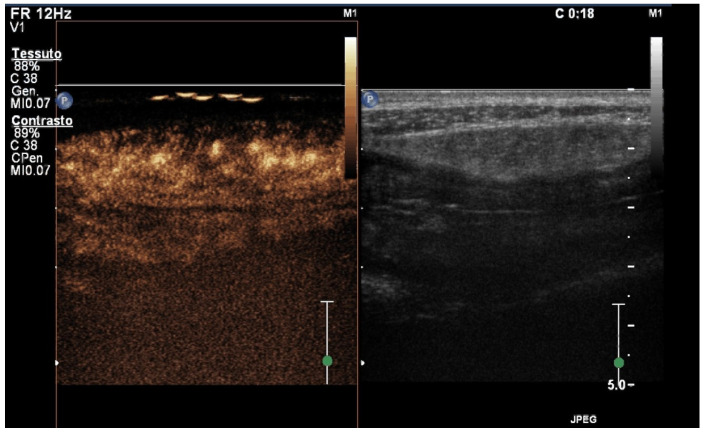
The terminal ileum presents a thickened and hypo-echoic bowel wall (left) with loss of normal layer stratification. Intestinal contrast-enhanced ultrasound (CEUS) image of the same tract showing thickened bowel wall and increased enhancement (right).
